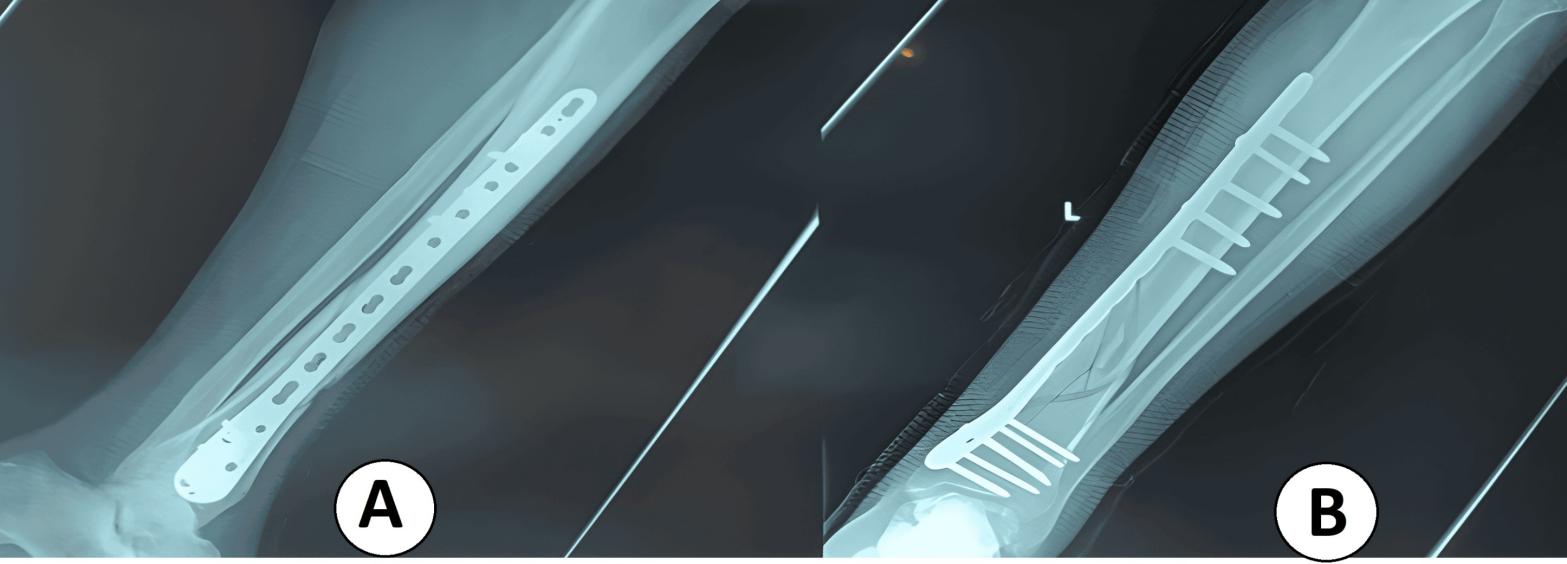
Immediate postoperative radiographs after MIPO locked plating of a distal tibial fracture (A) AP view: long bridging distal tibial plate spanning the metaphyseal–diaphyseal junction with clustered distal locking screws; alignment and length restored. (B) Lateral view: extra-articular plate position with appropriate screw lengths and maintained sagittal alignment; stable locked construct without joint penetration. MIPO: minimally invasive plate osteosynthesis.
